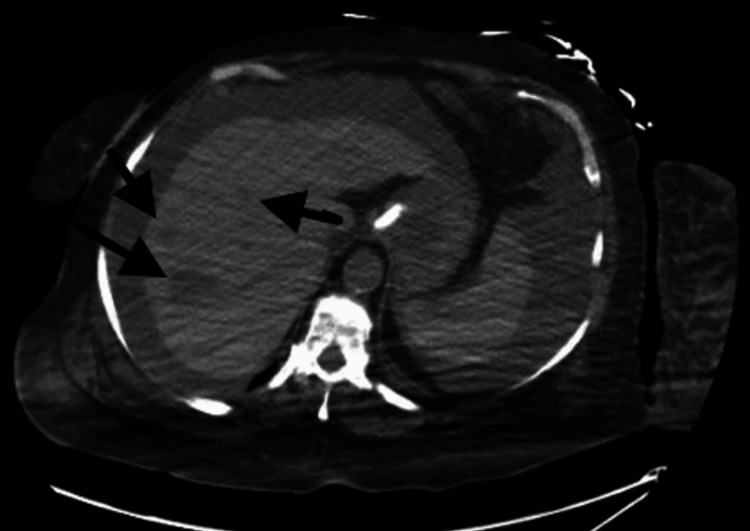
Computed tomography of the abdomen without contrast showing multiple right hepatic lesions.
